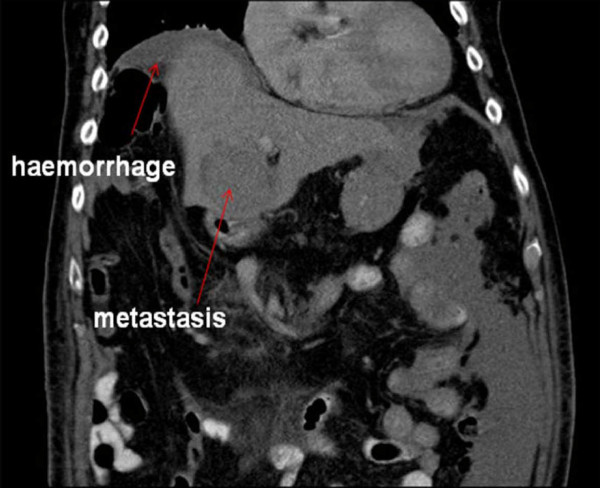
Coronal computed tomography view of the patient showing intra-abdominal hemorrhage and liver metastasis (red arrows).
